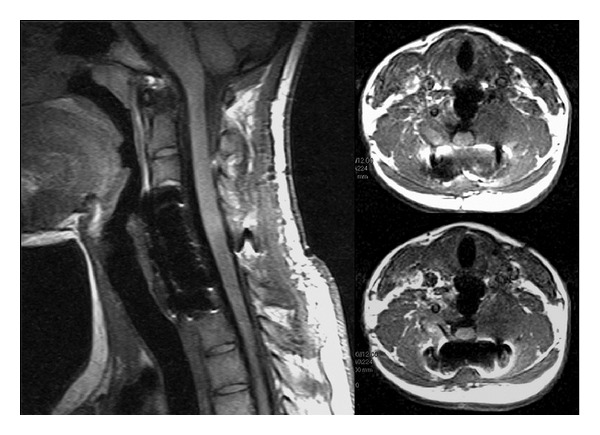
Six-month MRI scans, showing stability of the residual part of the lesion left around right vertebral artery.
